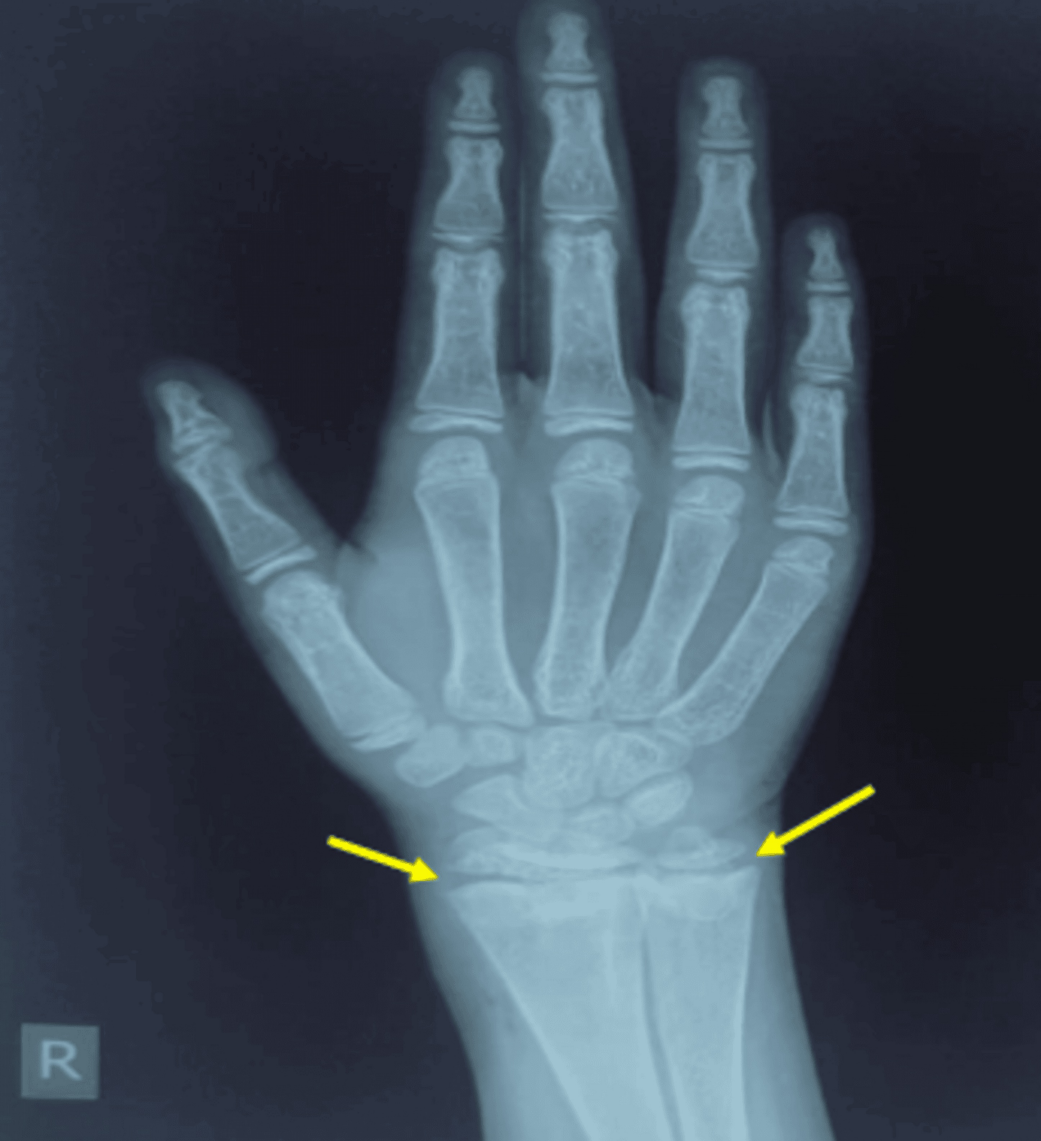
X-ray of left hand X-ray of hand at three-month follow-up showing provisional zone of calcification (yellow arrows).
